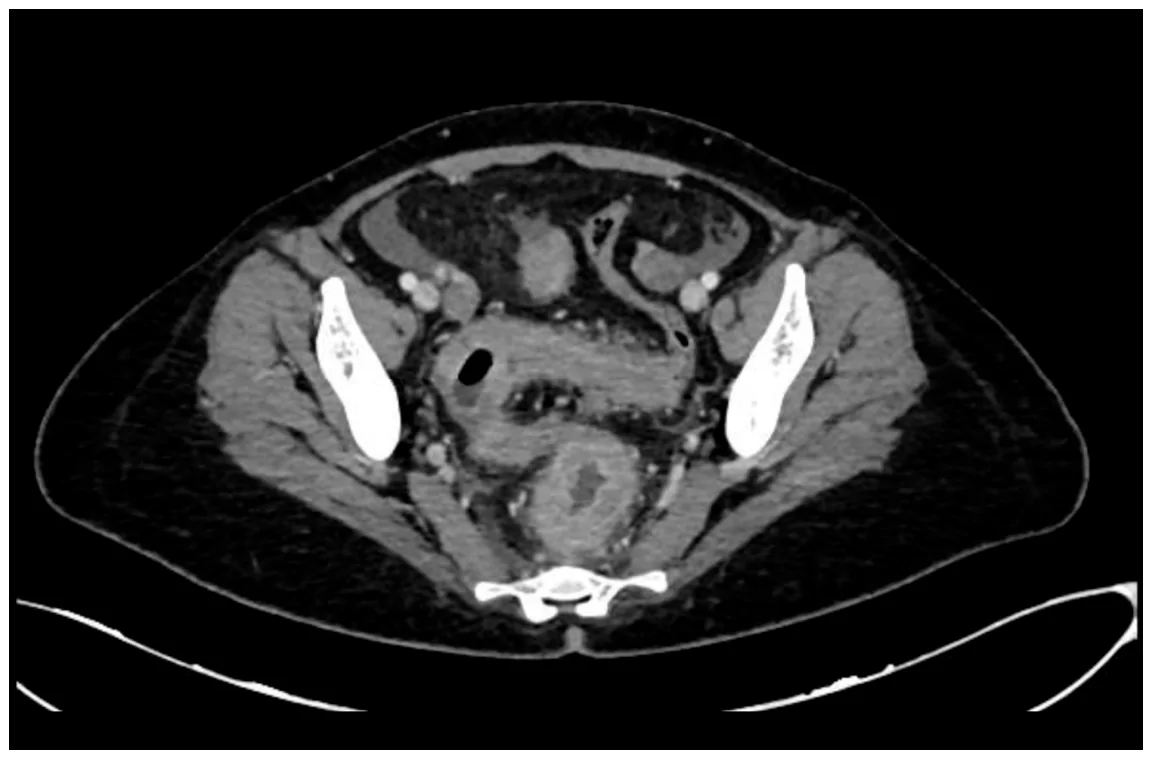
Initial contrast-enhanced CT of the abdomen and pelvis (axial). There is circumferential, multisegmental mural thickening of the large bowel with adjacent inflammatory fat stranding, an appearance initially attributed to inflammatory bowel disease.
